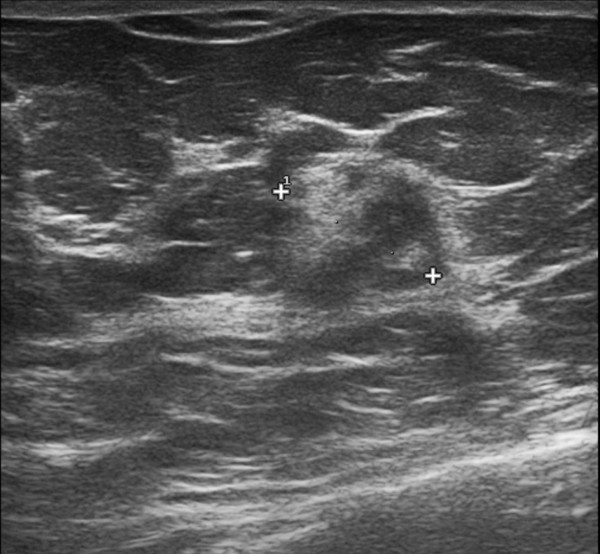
Ultrasound screening image. A corresponding heterogeneous mass shows increased echogenicity.
